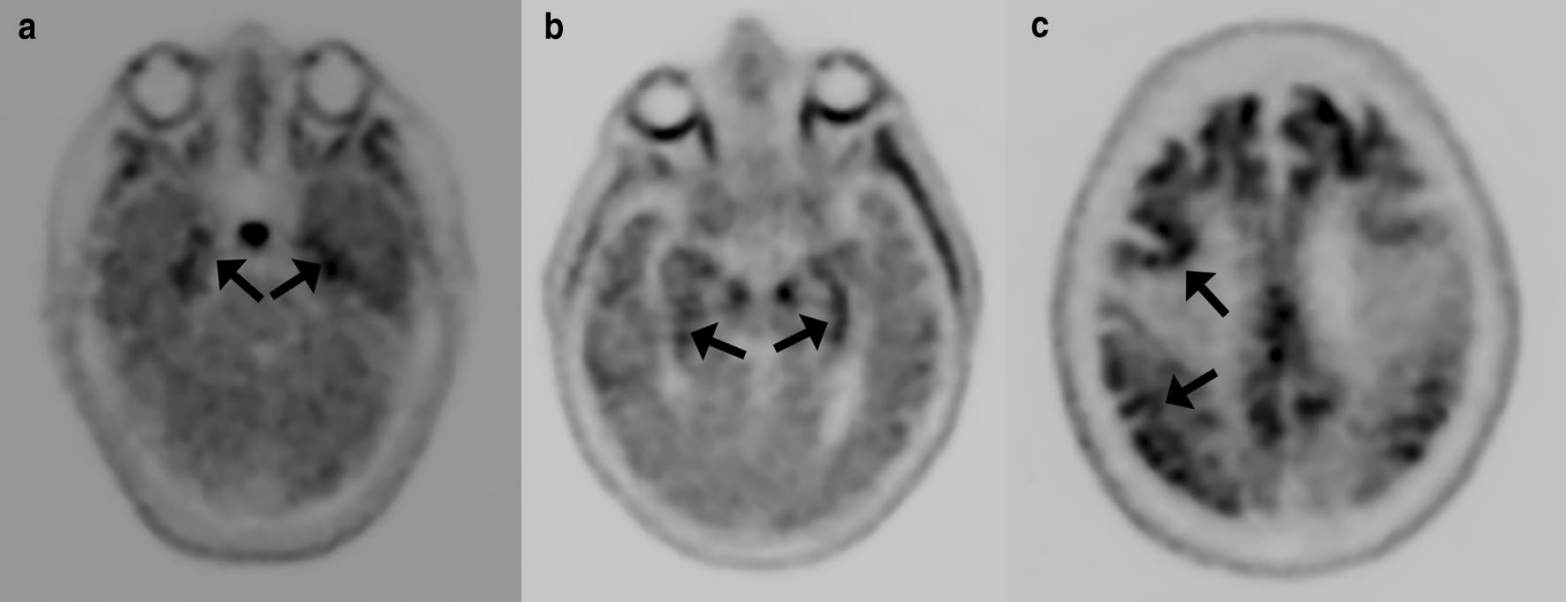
In vivo Braak staging on flortaucipir PET/CT. Activity confined to the entorhinal cortices and hippocampi falls into the Braak I-II stages (arrows in a), further progression in the temporal lobes and limbic cortices classifies stages III-IV (parahippocampal gyri; arrows in b), and neocortical activity defines stages V-VI (arrows in c)
